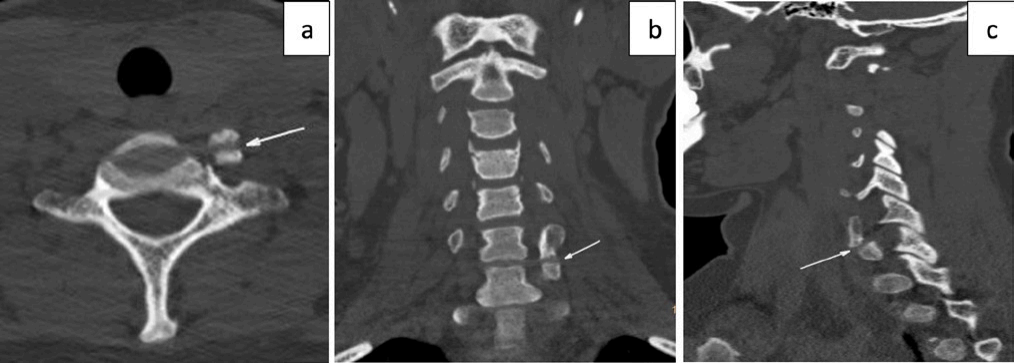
Cervical CT performed in the same patient with axial, coronal and sagittal reformations shows left-sided elongation of the anterior tubercle of the C6/C7 transverse process with an accessory articulation mimicking a fracture.
